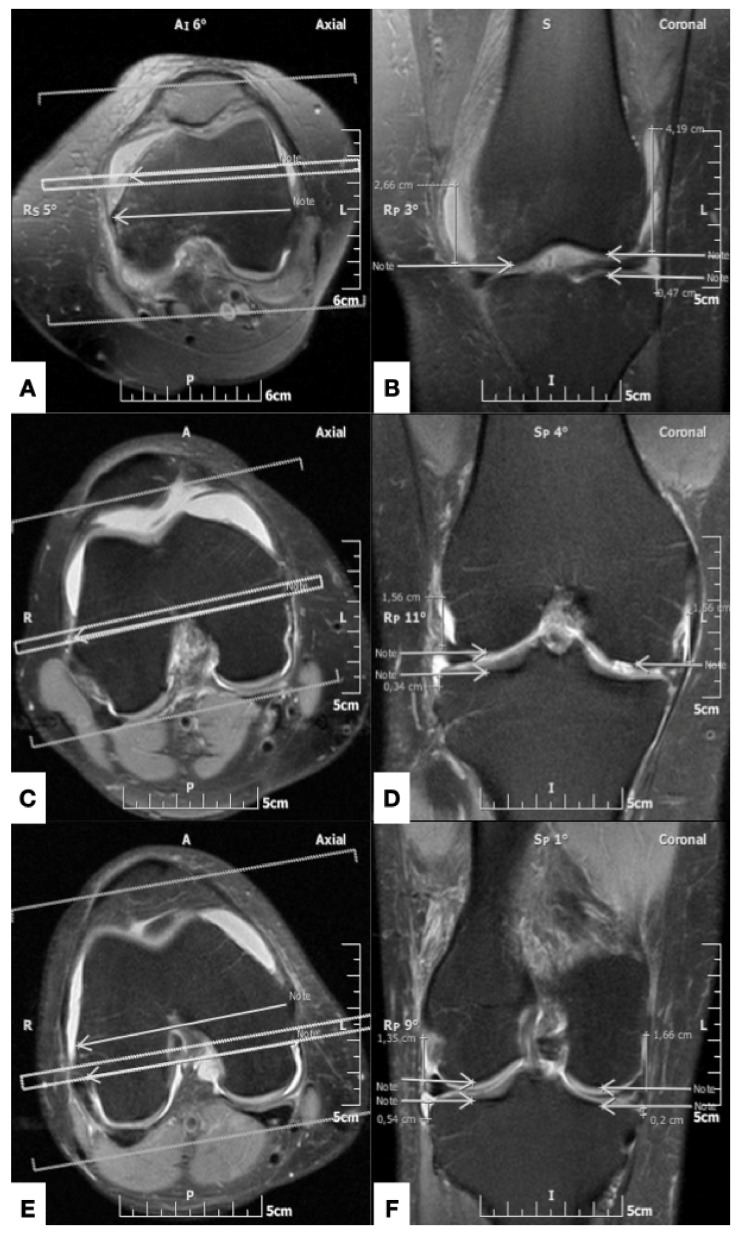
(A), (C) and (E), similar to Figure 2, shows the anterior, middle and posterior planes, respectively (double line in (A), (C) and (E), respectively), giving reference to the coronal section appearing in (B), (D) and (F). (B) Coronal fat-suppressed TSE intermediate-weighted MR image, shows the measurement of the medial femoral capsular reflection (distance between the left arrow and the superior margin of the knee intraarticular effusion), the lateral femoral capsular reflection (distance between the right top arrow and the superior margin of the effusion) and the lateral tibial capsular reflection (distance between the right bottom arrow and the inferior margin of the effusion), along the anterior plane. There is no measurable medial tibial capsular reflection on this plane. (D) Coronal fat-suppressed TSE intermediate-weighted MR image shows the measurements of the medial femoral capsular reflection (distance between the right arrow and the superior margin of the knee intraarticular effusion), the lateral femoral capsular reflection (distance between the left top arrow and the superior margin of the effusion) and the lateral tibial capsular reflection (distance between the left bottom arrow and the inferior margin of the effusion), in the middle plane. There is no measurable medial tibial capsular reflection on this plane. (F) Coronal fat-suppressed TSE intermediate-weighted MR image shows the measurements of the medial femoral capsular reflection (distance between the right top arrow and the superior margin of the knee intraarticular effusion), the lateral femoral capsular reflection (distance between the left top arrow and the superior margin of the effusion), the lateral tibial capsular reflection (distance between the left bottom arrow and the inferior margin of the effusion) and the medial tibial capsular reflection (distance between the right bottom arrow and the inferior margin of the effusion), along the middle plane.
